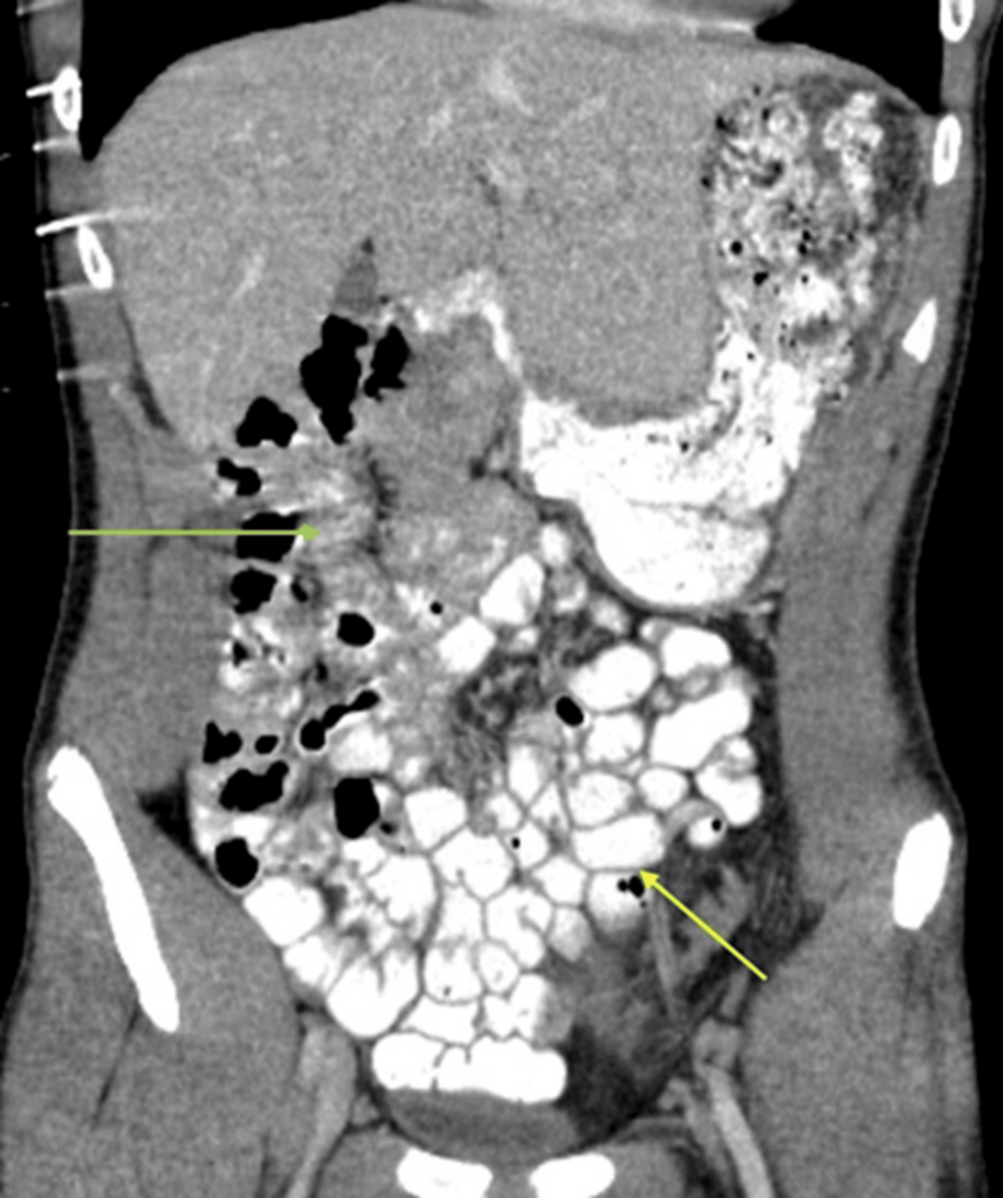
Coronal CT image of the abdomen This image shows the jejunal loops (green arrow) in the right upper abdomen and ileal loops (yellow arrow) in the left lower and mid abdomen.
